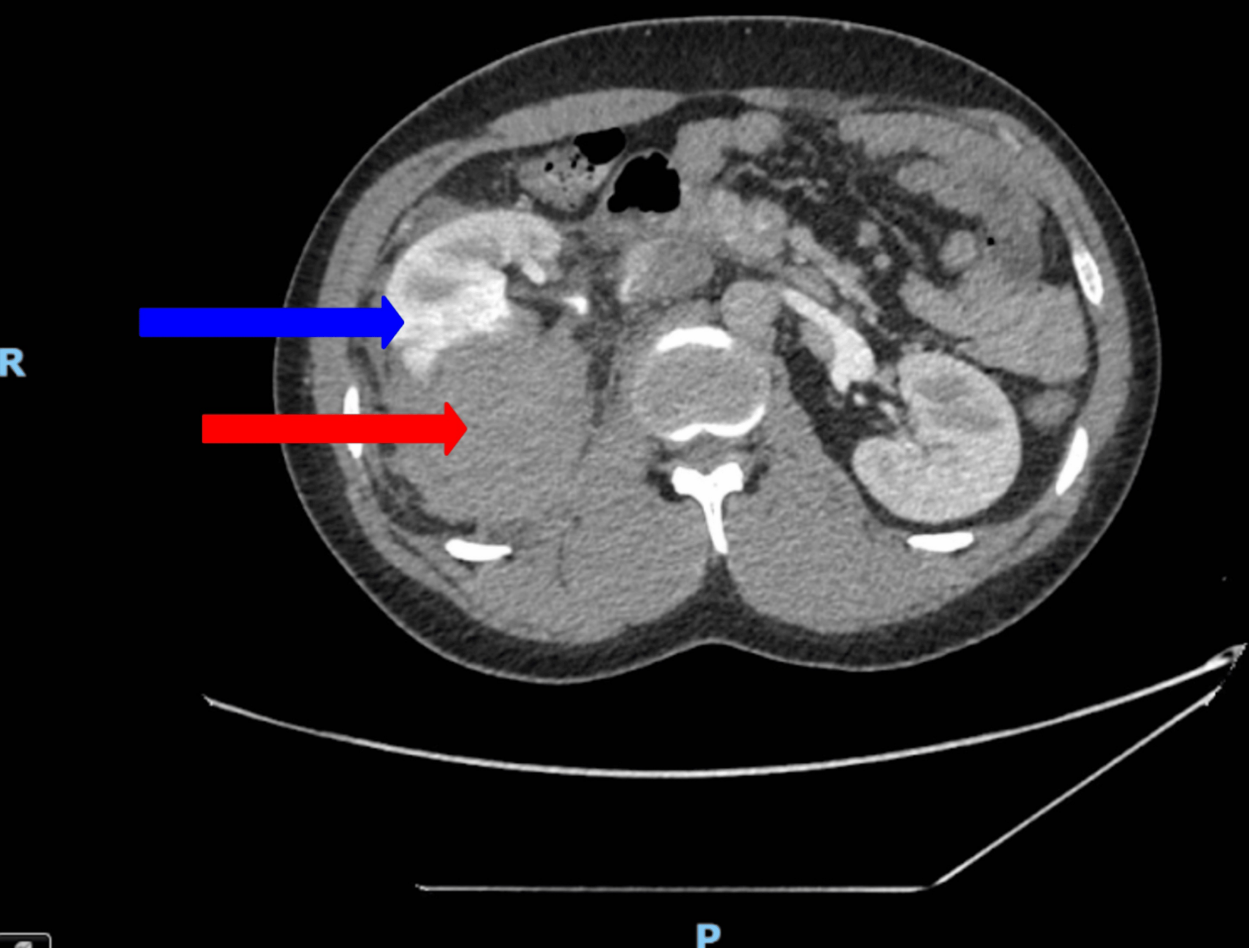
CT renal angiogram taken on day one of hospital admission The red arrow points to the stable perinephric hematoma. The blue arrow points to the shattered right kidney. The letter R depicts the right-hand-side of the patient. The letter P depicts the posterior surface of the patient.
